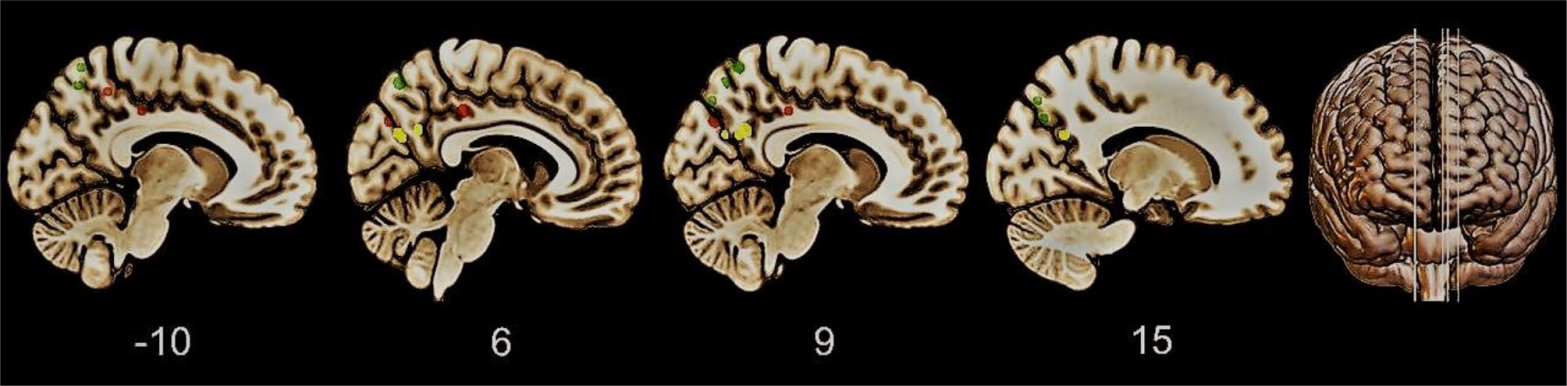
Graphical representation of the main PMC regions involved in visuospatial WM (yellow), awareness of memory deficits (red), and visuomotor control (green), i.e., posterior cingulate cortex (PCC) and precuneus (Pc). MRIcroGL software was used to circumscribe the ROIs on sagittal slices of T1-standard-template MRI. ROIs were extracted based on data from: Kochan et al. [17], Nobili et al. [69], Vannini et al. [50], and Karnath & Perenin [24]. Talairach coordinates were converted into MNI when necessary. MNI coordinates for visuospatial WM, PCC: *x* = 9, *y* = –57, *z* = 27; Pc: *x* = 6, *y* = –69 *z* = 24; *x* = 12, *y* = –60, *z* = 24. MNI coordinates for anosognosia for memory deficits, PCC: *x* = 6, *y* = –31, *z* = 38; *x* = –7, *y* = –29, *z* = 38; Pc: *x* = –7, *y* = –49, *z* = 49; *x* = 8, *y* = –74, *z* = 32. MNI coordinates for visuomotor control, Pc: *x* = –8, *y* = –64, *z* = 63; *x* = –8, *y* = –68, *z* = 53; *x* = 6, *y* = –70, *z* = 54; *x* = 7, *y* = –68, *z* = 54; *x* = 10, *y* = –60, *z* = 63; *x* = 13, *y* = –73, *z* = 44; *x* = 18, *y* = –70, *z* = 34
